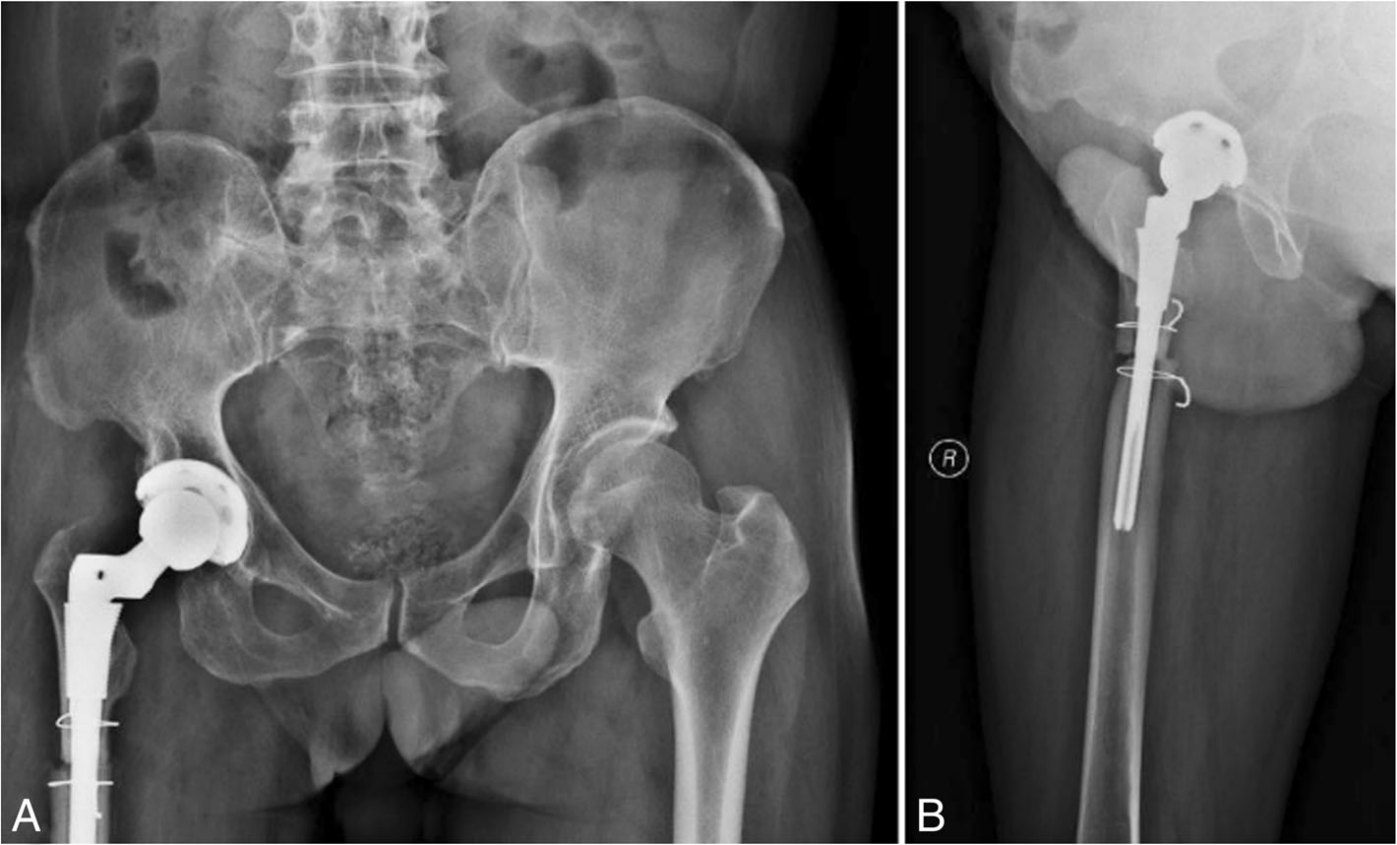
Radiographs of the right hip at three and a half months after the operation. (a) anteroposterior view of the right hip, (b) lateral view of the right hip
